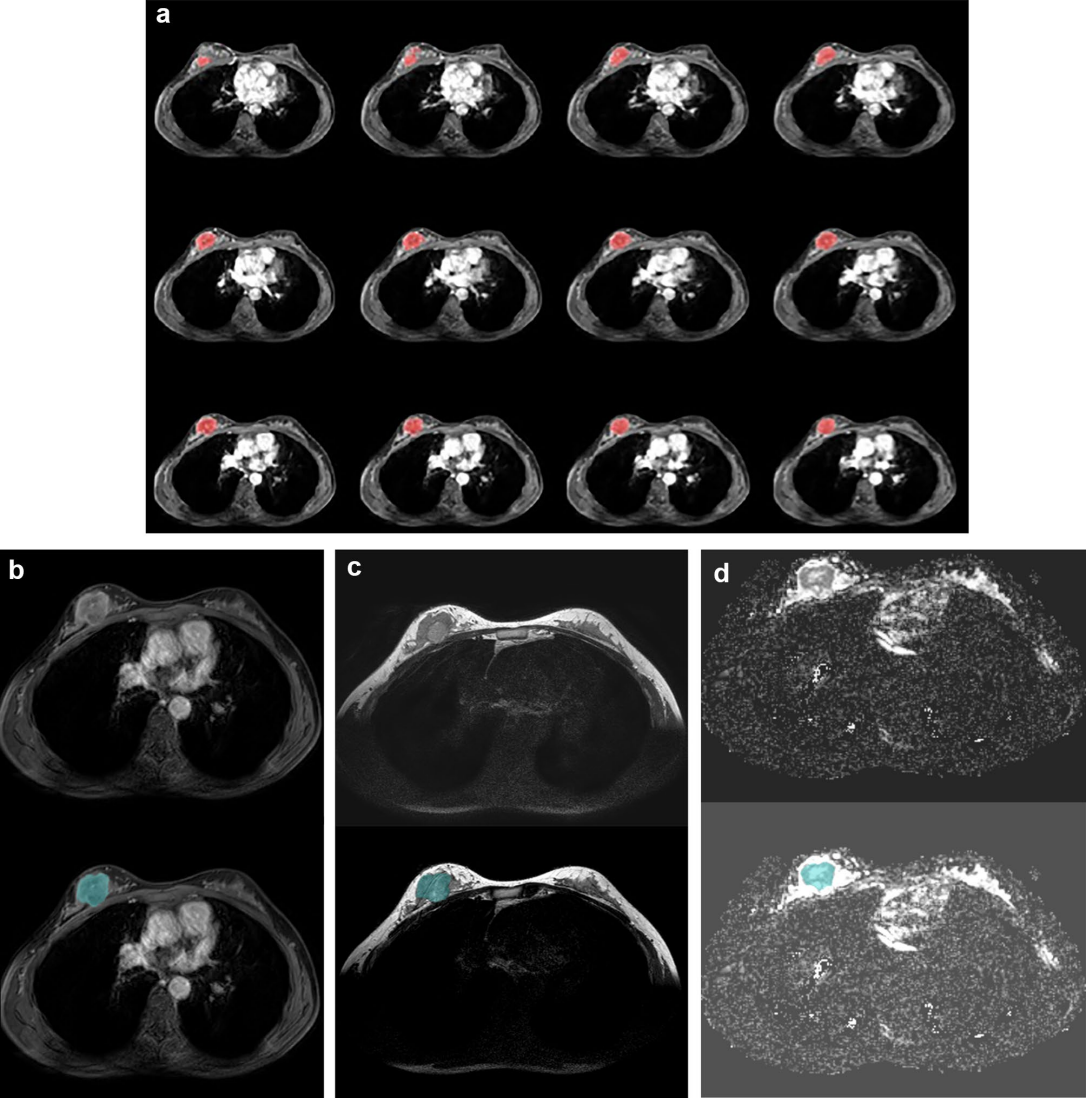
Texture analysis of invasive ductal carcinoma at 12 o’clock direction of right breast. Based on the VOI obtained from the perfusion parameters analysis (a), it was applied on subtracted early phase contrast enhanced T1WI (b), non-fat saturated T2WI (c), and ADC map (d). The color maps that do not match the tumor were manually modified using the original MR images of these sequences.
